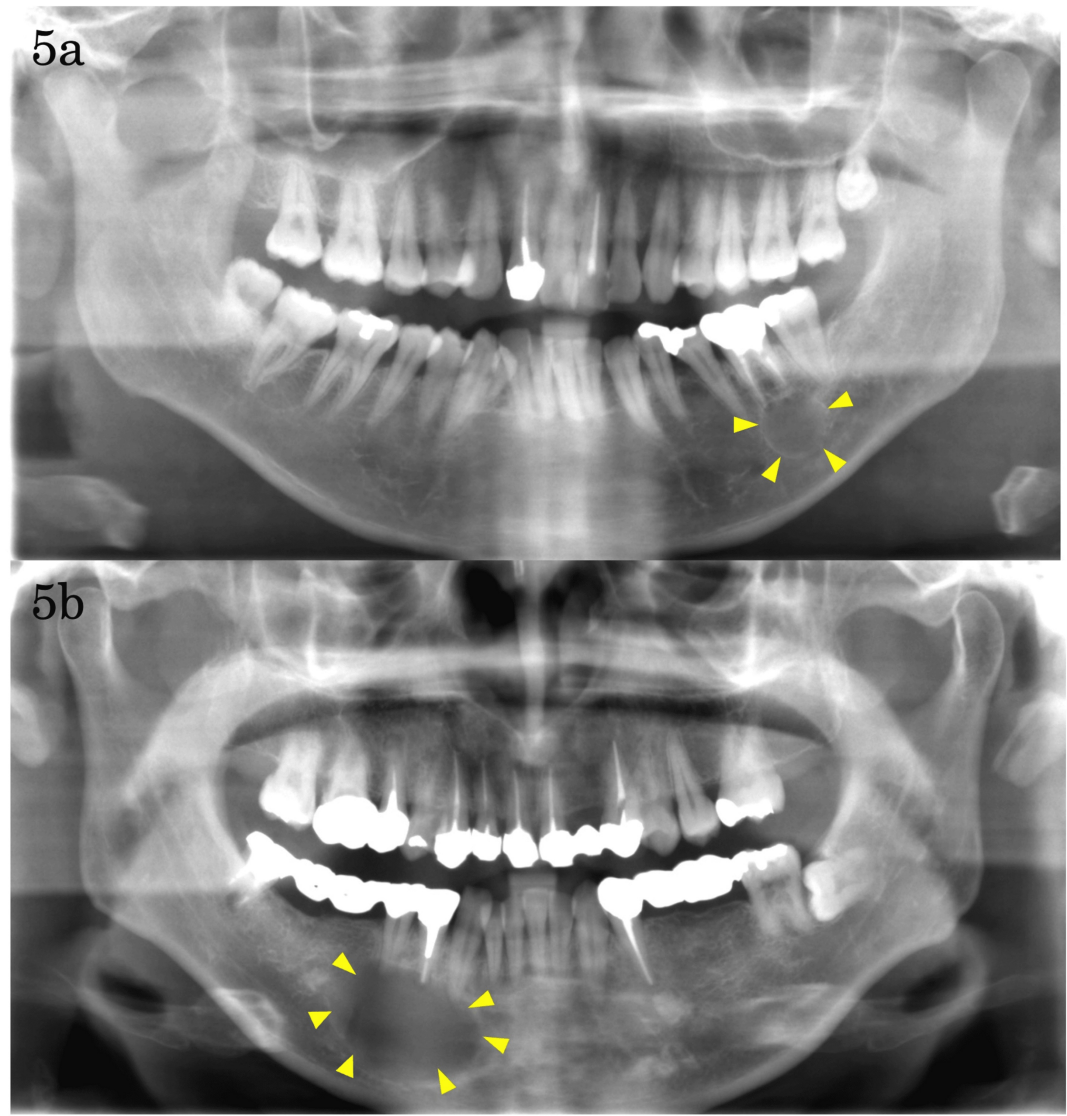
Assessment of lesion size using panoramic radiographs 5a: Cyst involving one or two teeth; 5b: Cysts involving three or more teeth.
